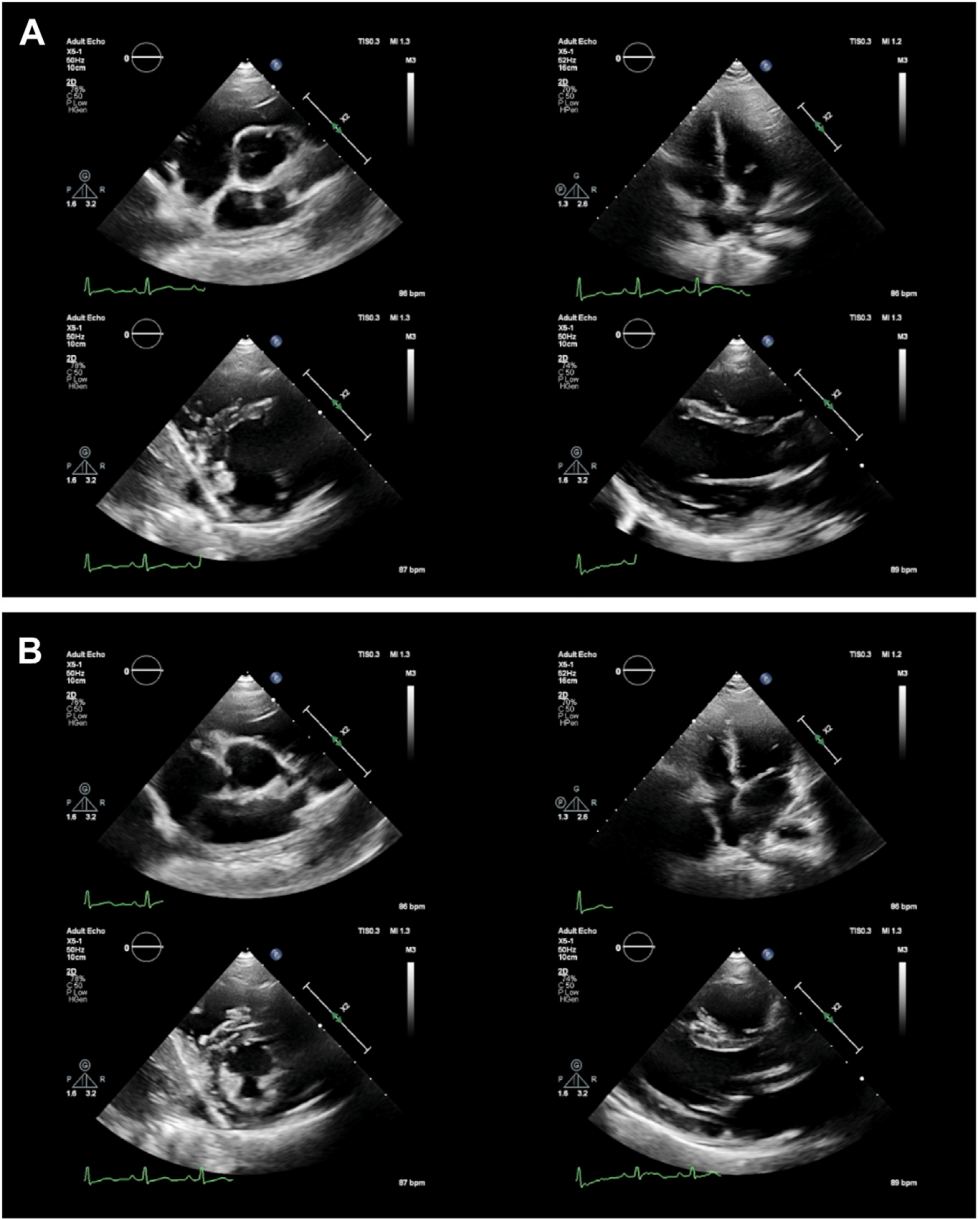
Two-dimensional TTE, basal parasternal short-axis (PSAX; *top left*), apical 4-chamber (*top right*), mid PSAX (*bottom left*), and parasternal long-axis (*bottom right*) views in (A) diastole and (B) systole reveal normal LV systolic function (LV ejection fraction 56%), normal wall thickness, and normal right heart dimensions.
